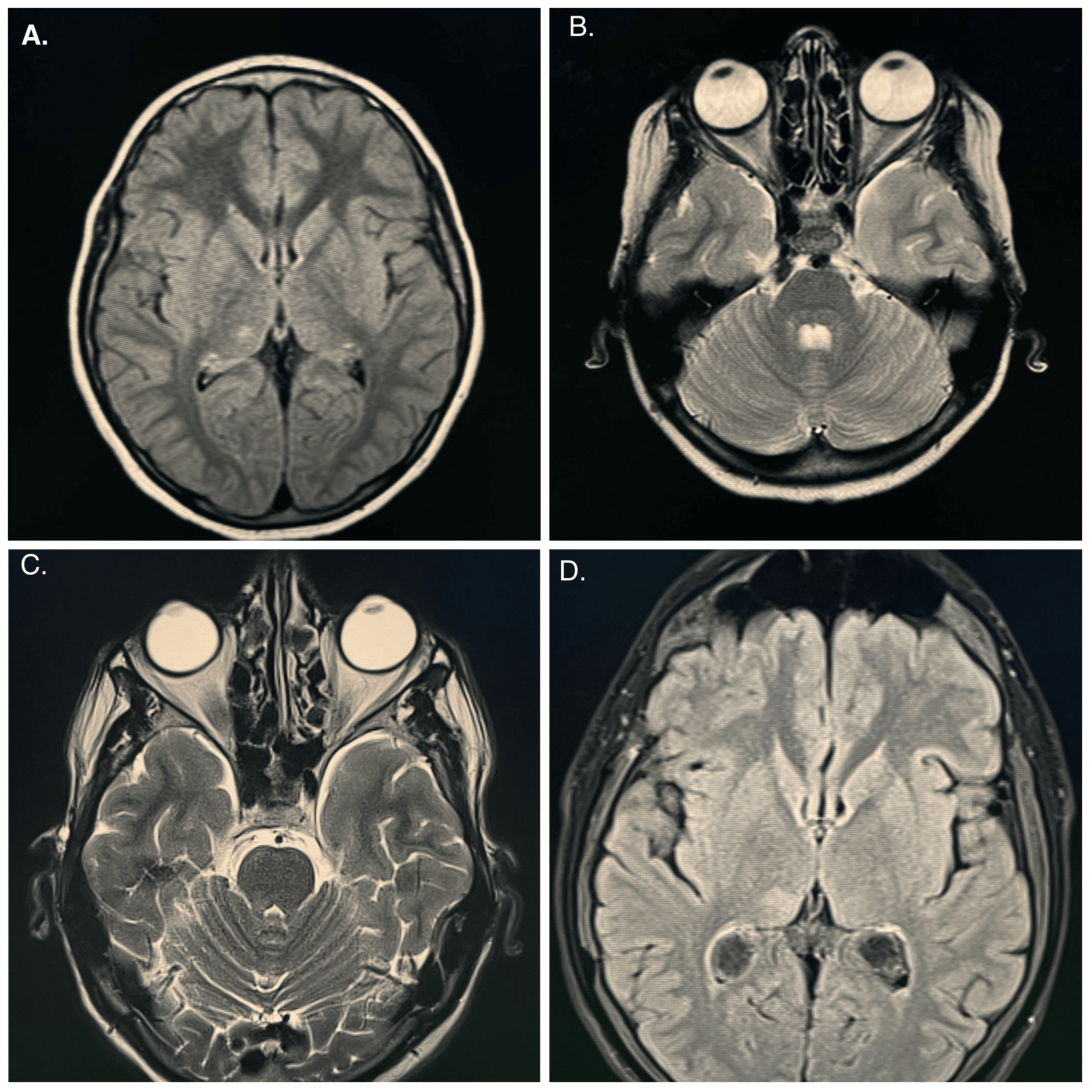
Axial fluid-attenuated inversion recovery (FLAIR) MRI brain images from 2012 (A-B) and 2025 (C-D) showing a stable, non-enhancing hyperintense lesion in the right thalamus, consistent with a long-standing benign structural finding.
